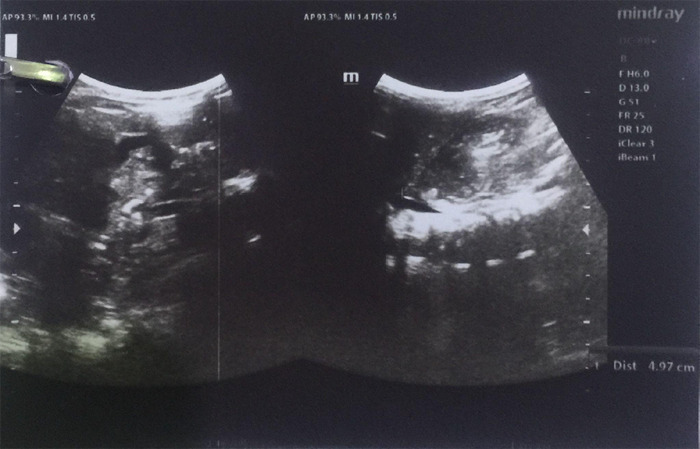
Cross-fused renal ectopia as seen on ultrasonography.
